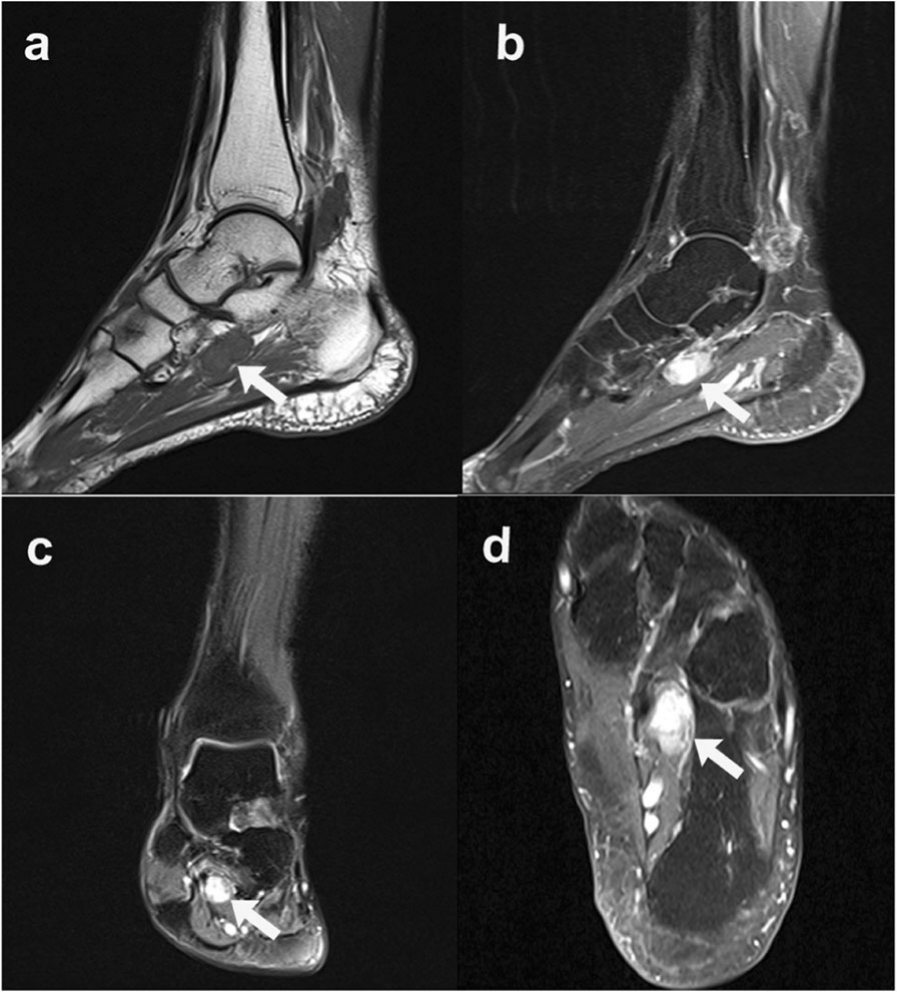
Magnetic resonance images of the patient’s left foot. a Sagittal T1-weighted turbo spin echo (TSE) image. b Sagittal T1-weighted TSE contrast-enhanced image with Ga-DTPA fat -suppressed. c Coronal proton density (PD)-weighted TSE fat-suppressed image. d Transverse T1-weighted TSE contrast-enhanced image with Ga-DTPA fat-suppression. Another well-enhanced mass was identified beside the flexor hallucis longus tendon at the level of the talonavicular joint (arrows). Considering that the patient was diagnosed with melanoma, these appearances were in favor of metastases from melanoma according to a radiologist. However, the differential diagnosis was pigmented villous nodular synovitis
